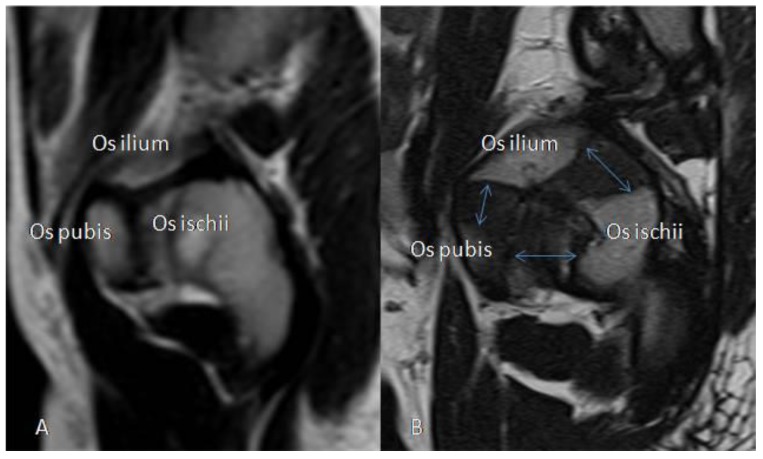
Magnetic resonance imaging (MRI) presentation of a widened tri-radiate cartilage of the acetabulum. MRI (T2 weighted, sagittal) of the tri-radiate cartilage of the left acetabulum (A) in a 5.6-year-old healthy male and (B) in a 6.7-year-old female ML intermediate patient (*n* 16) with widening of the cartilage (blue arrows).
